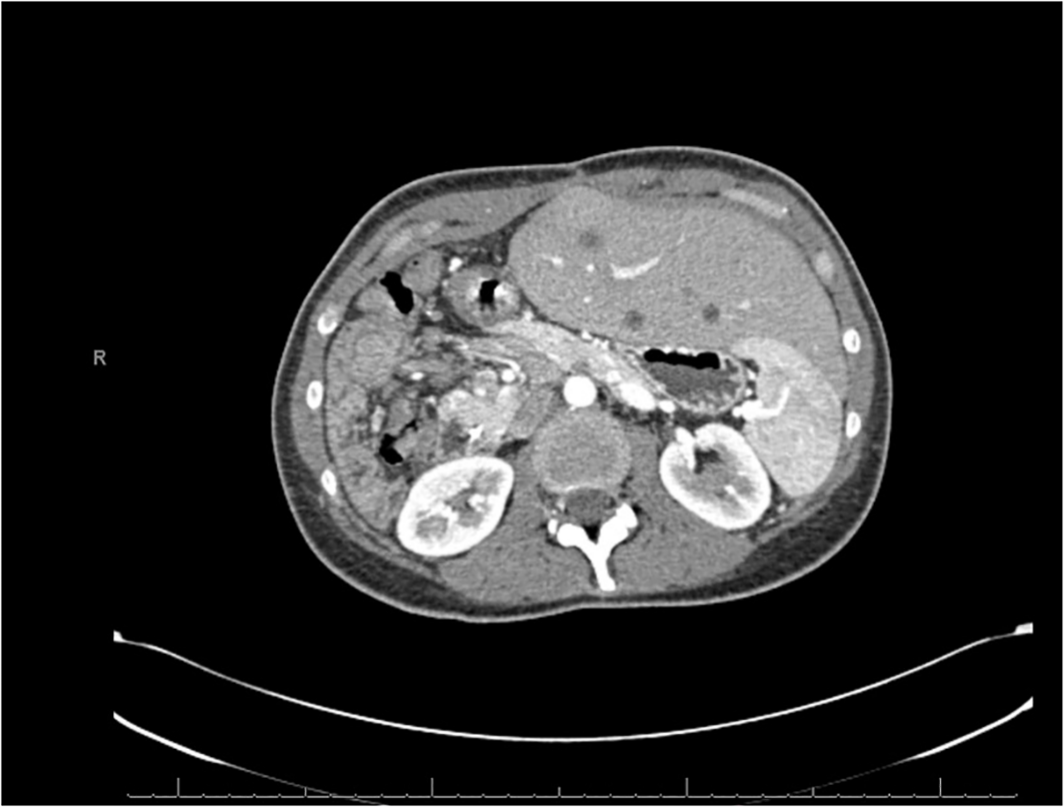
CT scan dated July 2022 showing recurrent lesions in the liver. The left lobe of the liver has grown significantly to compensate for the prior hepatic resection.
